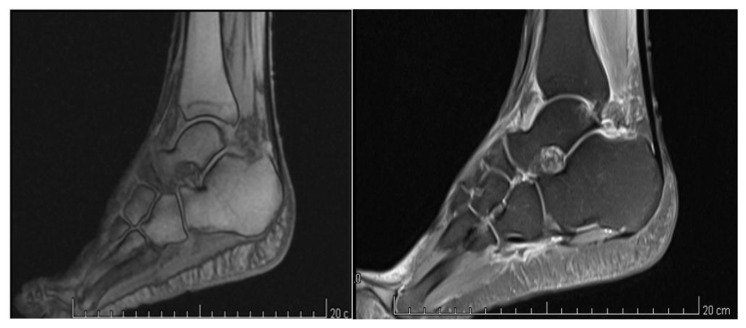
MRI of the right ankle and foot of the patient showing soft tissue edema without bone involvement.
